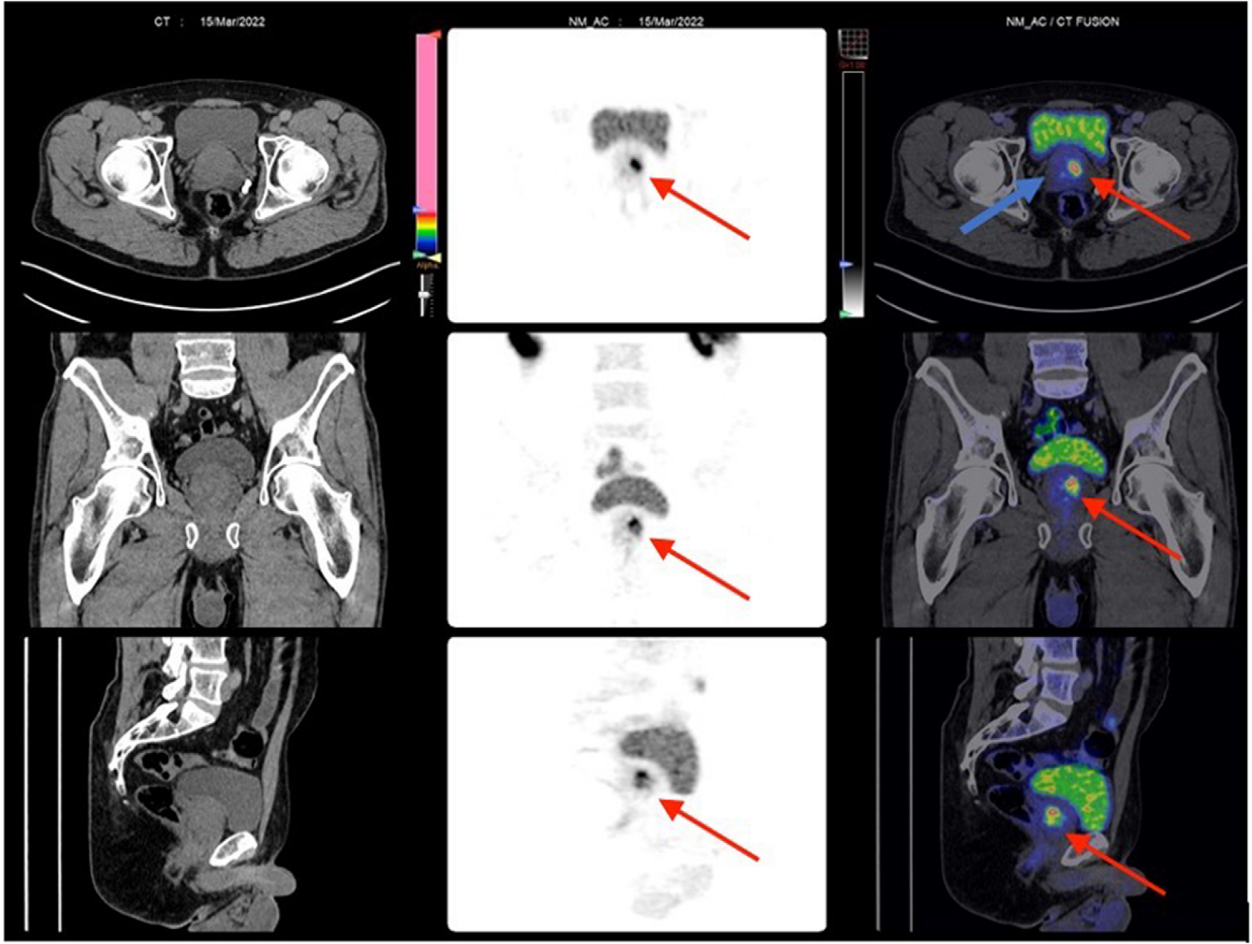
PSMA PET CT. Red arrows identify areas of the prostate (left prostatic base) that had increased uptake. Right base where the TRUS biopsy had been positive does not show any PSMA uptake (blue arrow).
